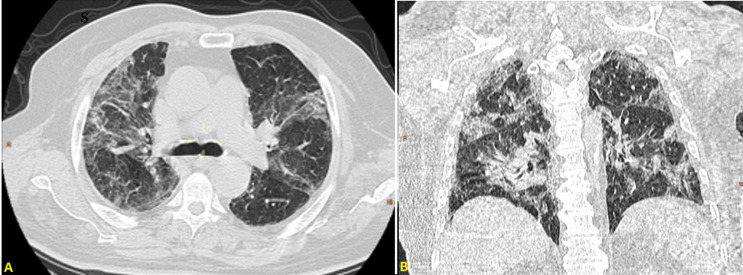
thoracic CT scan in an axial (A); and coronal (B) window showing 60% of CT abnormalities related to for peer review COVID-19 disease
